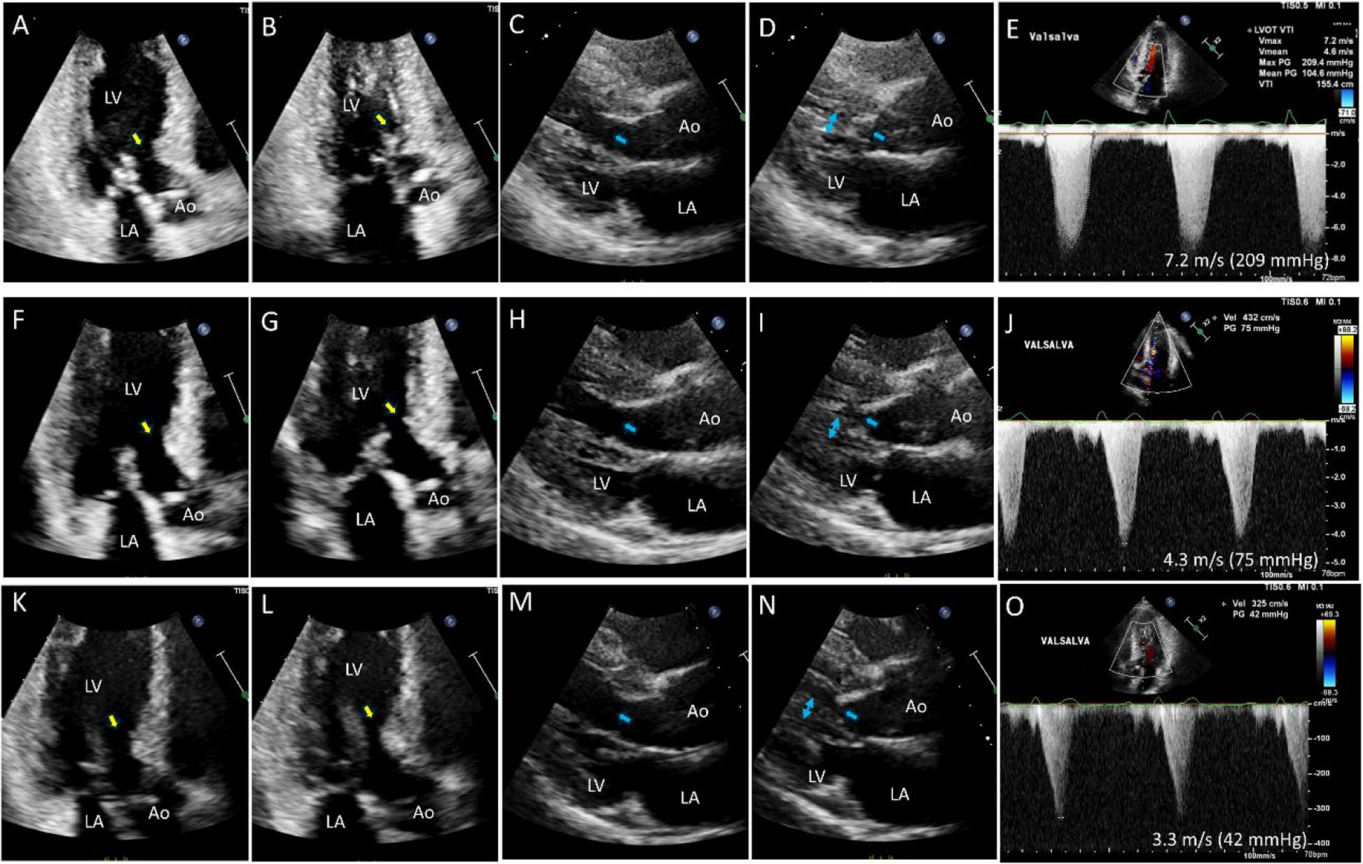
A representative case showing improvement of multiple mechanisms of left ventricular outflow tract obstruction with administration of a cardiac myosin inhibitor. (A, B) A 67-year-old man complained of severe effort dyspnea after a successful percutaneous coronary intervention. Echocardiography showed prominent systolic anterior motion of the mitral valve (SAM) with septal contact. (C, D) Elongated papillary muscle also contributed to (E) left ventricular outflow tract (LVOT) obstruction. At 1 month of administration of a cardiac myosin inhibitor, (F, G) SAM-septal contact was lost, but (H, I) septal contact of the elongated papillary muscle persisted, and (J) the peak velocity of LVOT flow decreased significantly. Three months after medication, (K–N) both SAM and elongated papillary muscle-septal contact were absent, which resulted in (O) marked decrease in LVOT peak velocity
